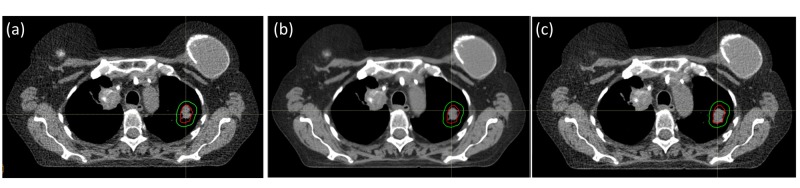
Target contour definition The ITV (green) and IGTV (red) contours as observed in the (a) maximal inspiration phase, (b) average CT scan and (c) maximal expiration phase of the breathing cycle. Note that the IGTV has been contoured directly on the MIP and transferred to the individual breathing phase CTs. ITV: internal target volume, IGTV: internal gross target volume, MIP: maximum intensity projection
